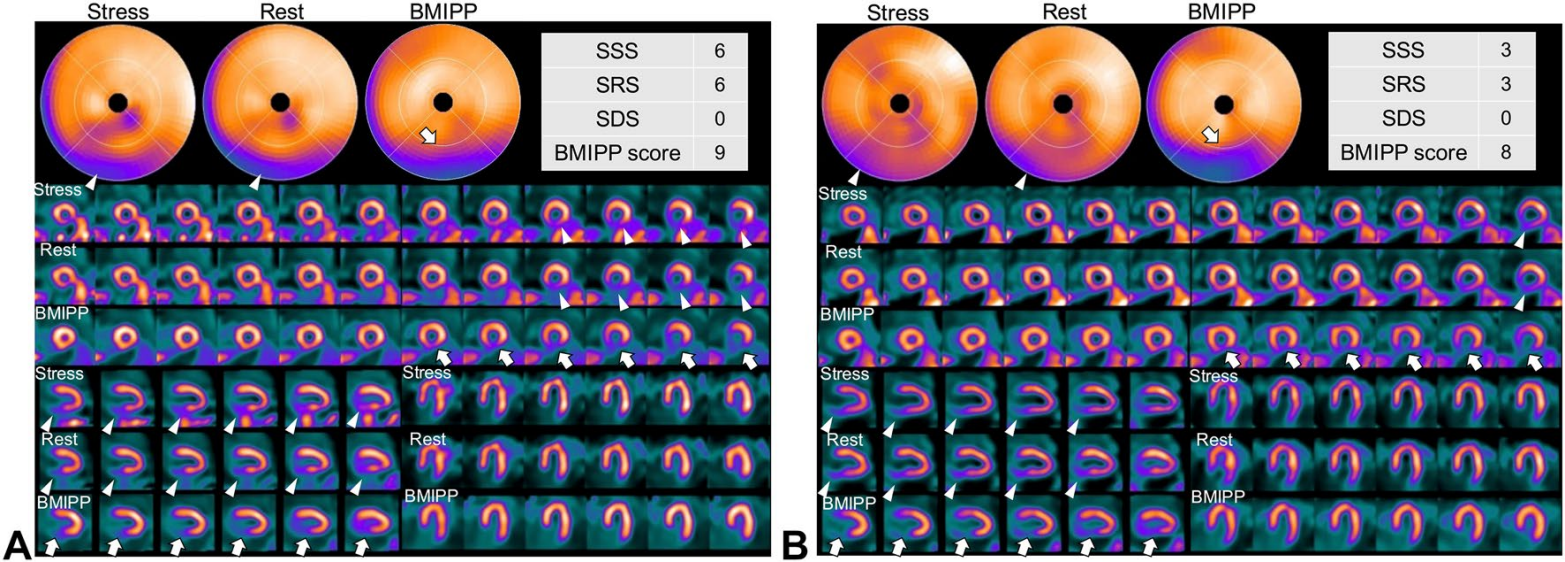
a At 17 months after CABG. ^99m^Tc-tetrofosmin pharmacological stress perfusion SPECT showed inferoposterior infarction as scored SRS 6 (white arrow head) and no ischemia. In BMIPP SPECT, there was a severe decrease to complete defect in the inferior wall with a perfusion–metabolic mismatch (white arrow) as scored BMIPP score 9. b BMIPP SPECT at 47 months after CABG showed a persistent defect in the inferior wall with a perfusion–metabolic mismatch as scored BMIPP score 8 (white arrow), while a perfusion defect was improved and scored SRS 3 (white arrow head)
